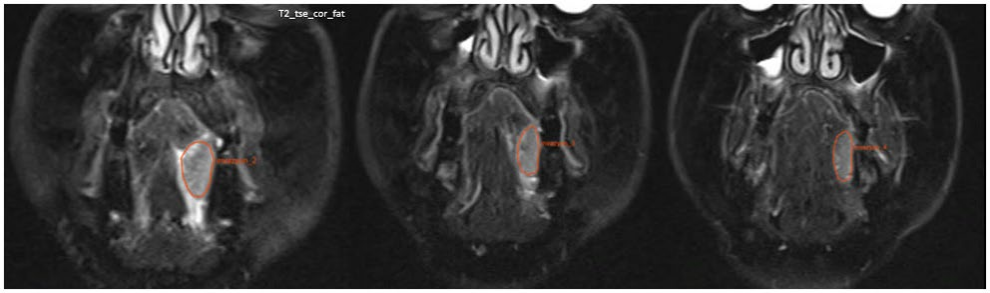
T2-TSE-coronal-fat-suppressed MRI images showing the oral SCC with manual delineation on consecutive MR slices
